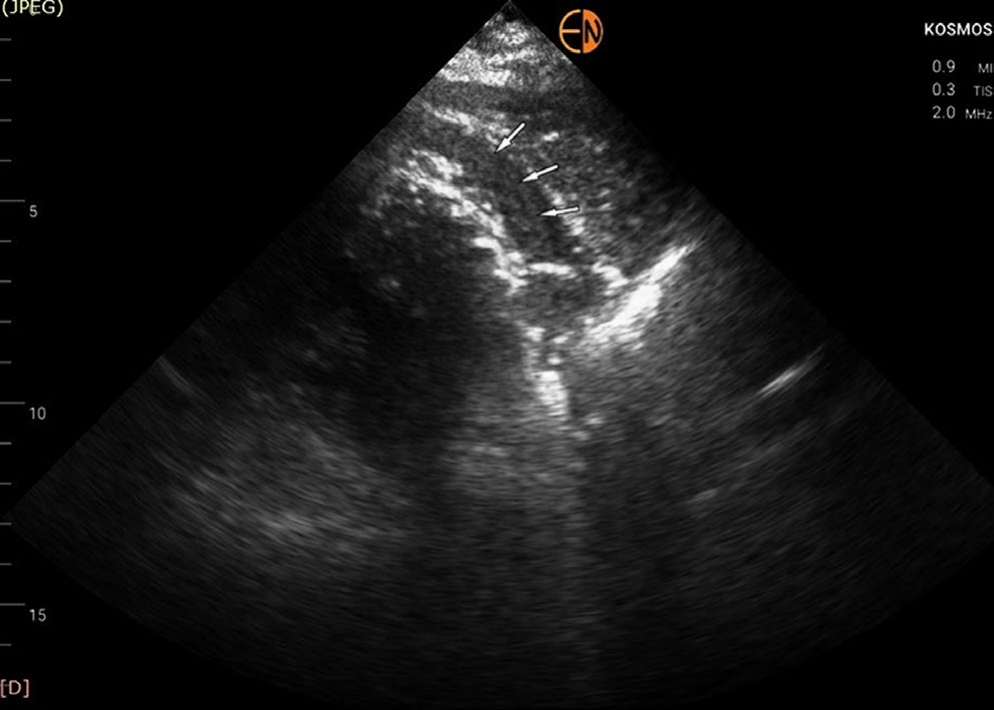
Modified parasternal short‐axis view showing the presence of intrapericardial hematoma (white arrows)
